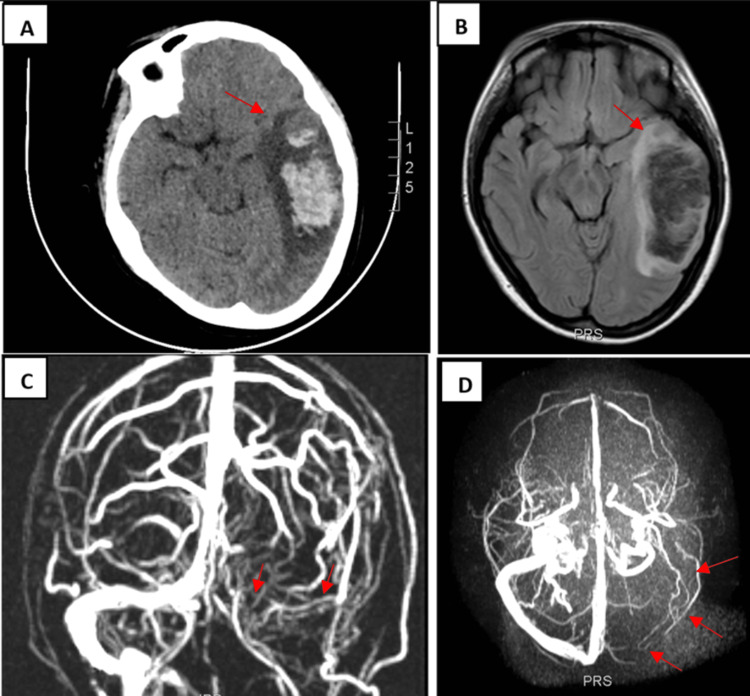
Non-contrast CT scan, FLAIR, and MRV images. Non-contrast CT scan of the head showed a left parietotemporal intraparenchymal hemorrhage with surrounding edema (red arrow) (A). FLAIR showed intraparenchymal hemorrhage with edematous changes (red arrow) (B). MRV images showed an absence of filling of the left transverse sinus (red arrows) (C, D). CT: computed tomography, FLAIR: fluid-attenuated inversion recovery, MRV: magnetic resonance venography.
